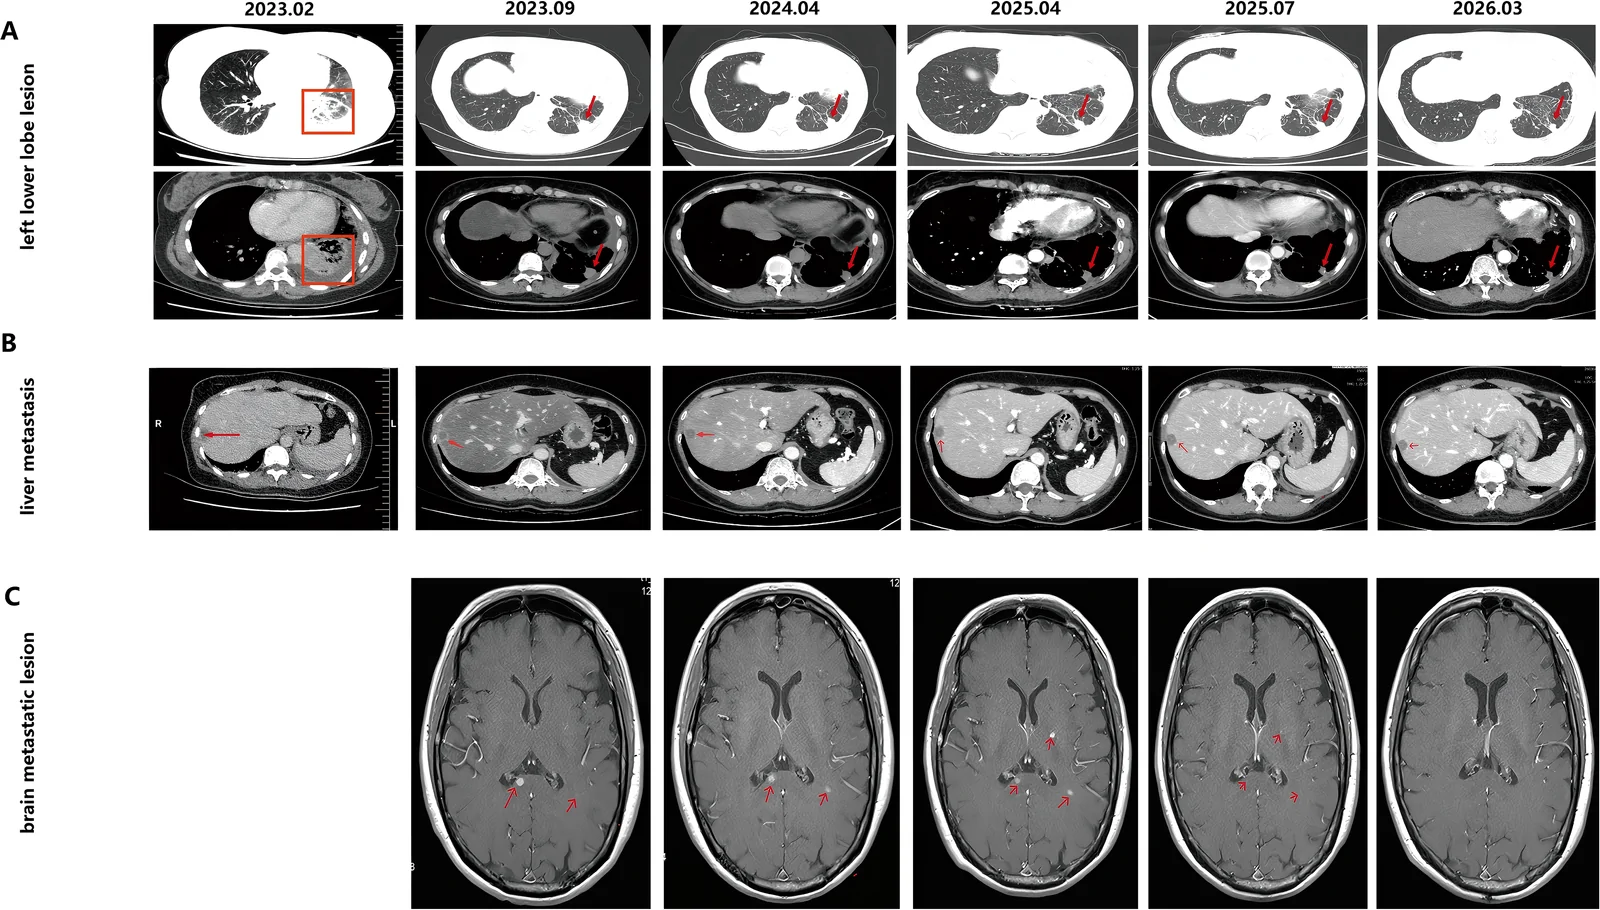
Serial radiological imaging monitoring of target lesions across all disease sites from 2023 to 2026. (A) Left lower lobe primary lesion: Upper panels represent lung window CT scans; lower panels show mediastinal window CT scans, with red arrows marking the primary tumor. (B) Hepatic metastases: Abdominal CT slices with red arrows indicating liver metastatic lesions, which remained stable throughout ensartinib treatment. (C) Intracranial metastases: Brain MRI sequences displaying cerebral metastatic foci (red arrows), which achieved complete radiographic resolution by March 2026 follow-up.
